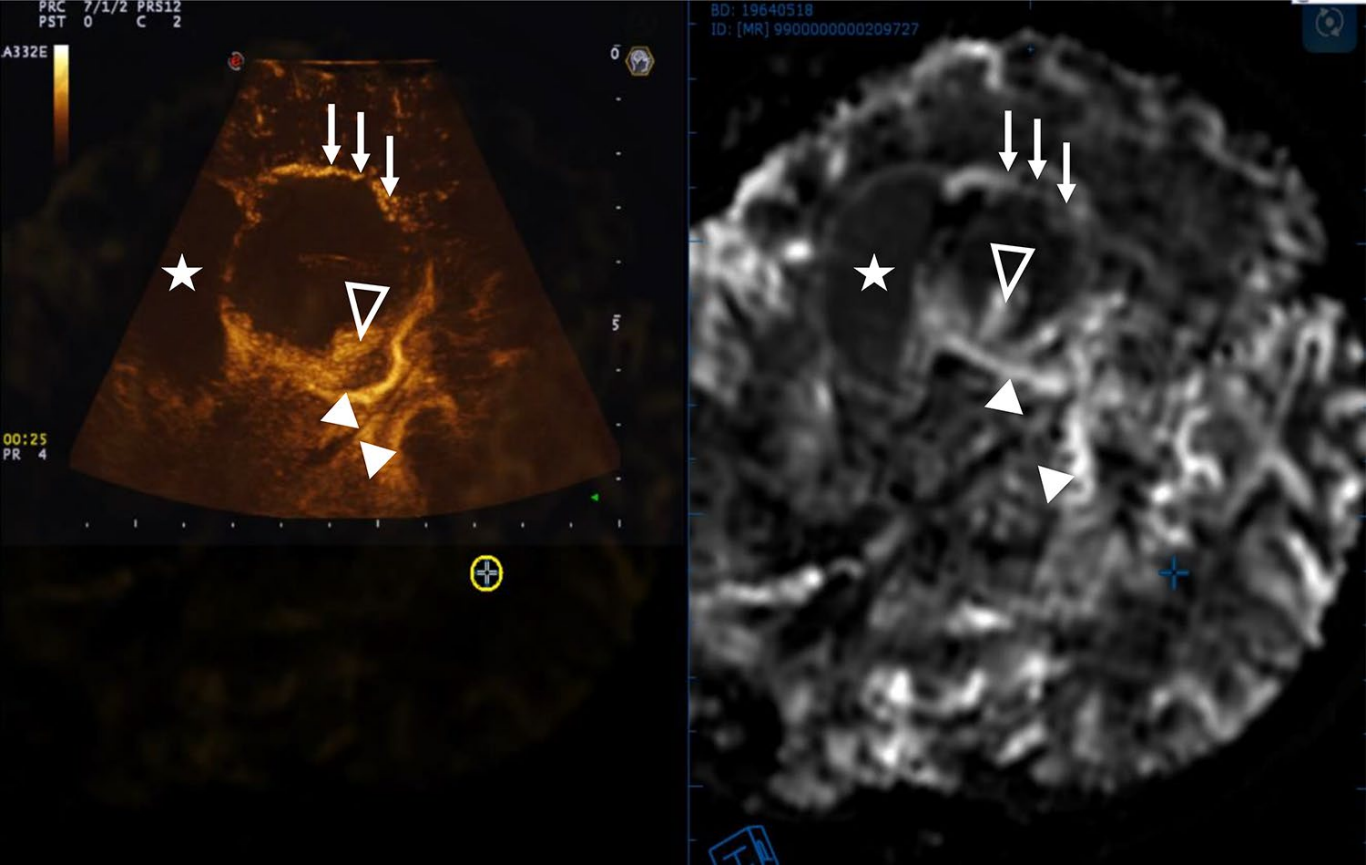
Fusion imaging and navigation between direct cerebral CEUS imaging and MRI perfusion imaging: CEUS image is displayed on the left of the screen coupled with the co-planar MRI on the right side. The two imaging modalities are linked together and the MRI follow the real-time CEUS modality as the US probe is tracked in the 3D space. From a qualitative standpoint MBs distribution grossly follows Gadolinium distribution on MRI as visible with anatomic landmarks: three white arrows, tumor periphery—star, tumor necrotic center—empty arrowhead, tumor bulk—double arrowheads, internal cerebral veins.
